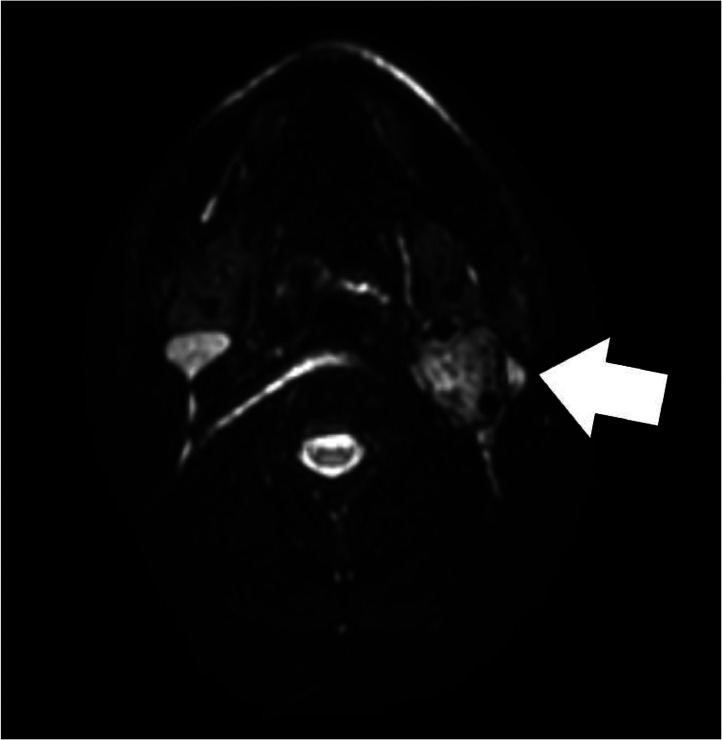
STIR DWI axial MRI neck depicting well-defined lesion in left carotid triangle showing restriction. Legend: STIR, Short tau inversion recovery; DWI, Diffusion weighted imaging; MRI, Magnetic resonance imaging.
